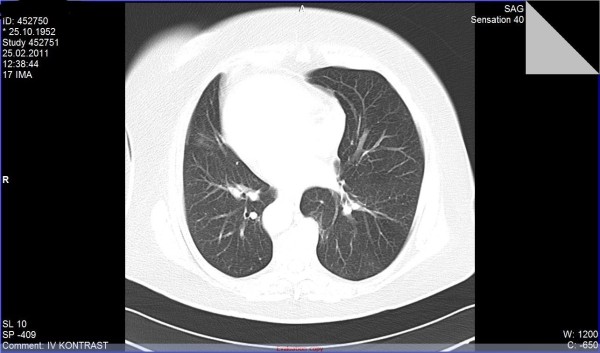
Thoracic computed tomography scan. It shows dextrocardia and scars of previous pulmonary infections.
